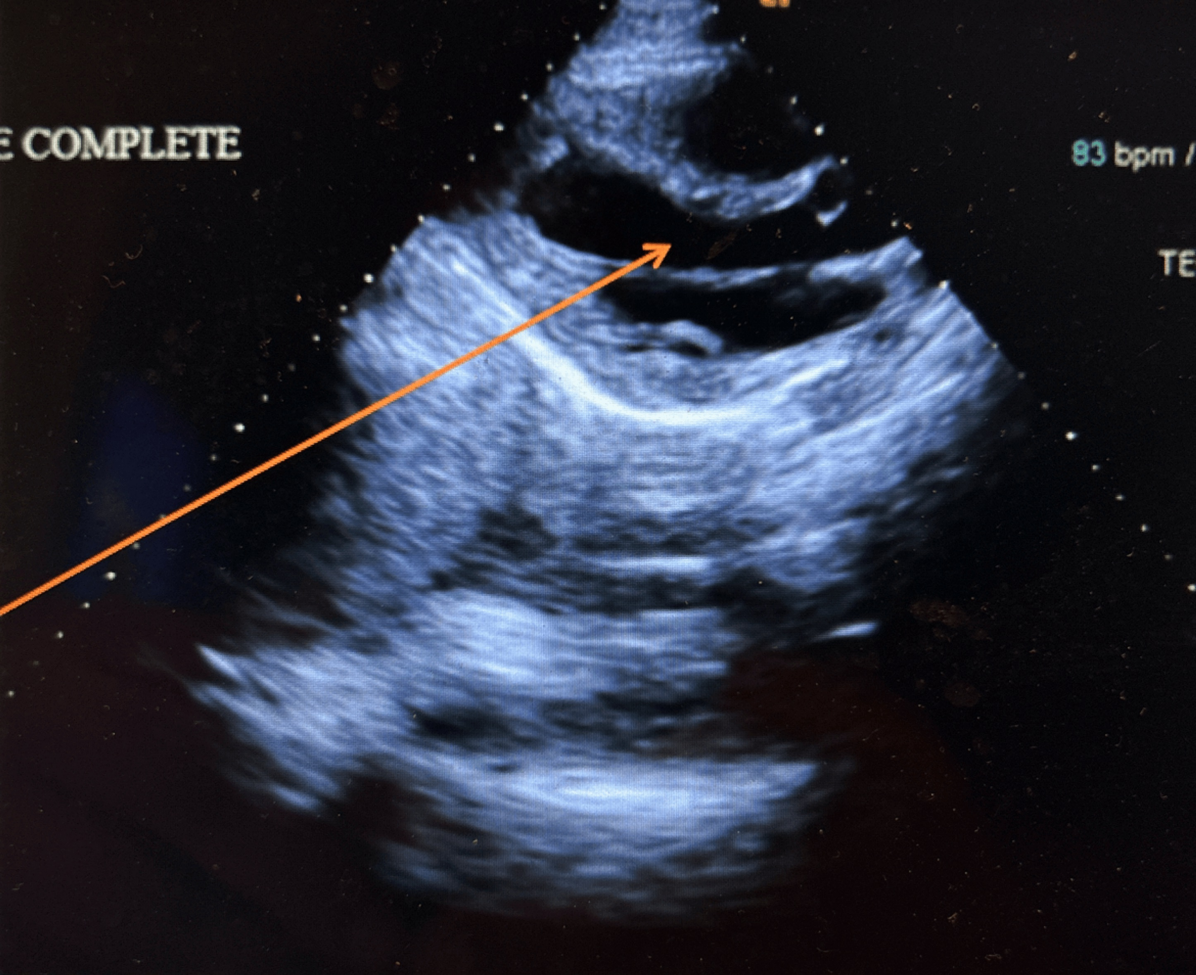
Echocardiogram showing no evidence of pericardial effusion.
